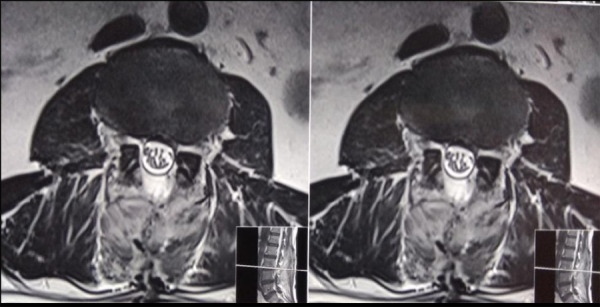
Postoperative 3 Tesla MRI LS spine T2 weighted axial section at the L3-L4 level.
